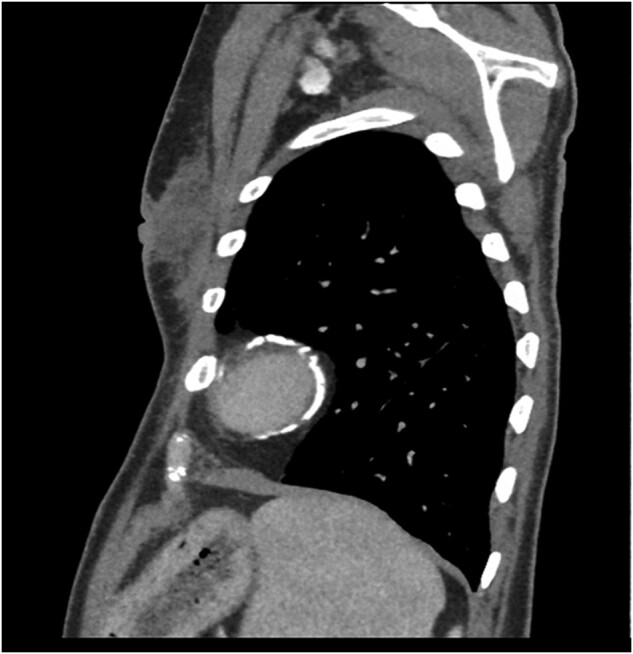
Sagittal view of computed tomography thorax demonstrating circumferential calcification of the apical region of left ventricle.
